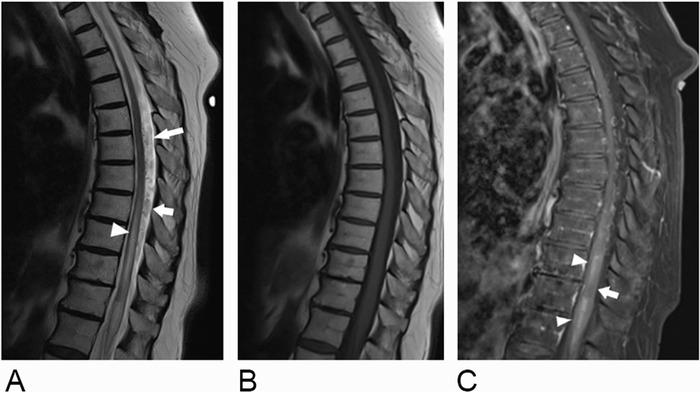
Magnetic resonance imaging of the thoracic spine. (A) Sagittal T2-weighted image through the level of the mid-thoracic spinal cord demonstrates long segment central cord hyperintensity and expansion in the lower thoracic cord (arrowhead) and prominent serpentine T2 flow voids in the posterior intradural space (arrows). Sagittal T1-weighted images (B) before and (C) after contrast administration with fat saturation demonstrate corresponding patchy central cord enhancement (arrowheads) with a discrete nonenhancing segment known as the missing-piece sign (arrow).
